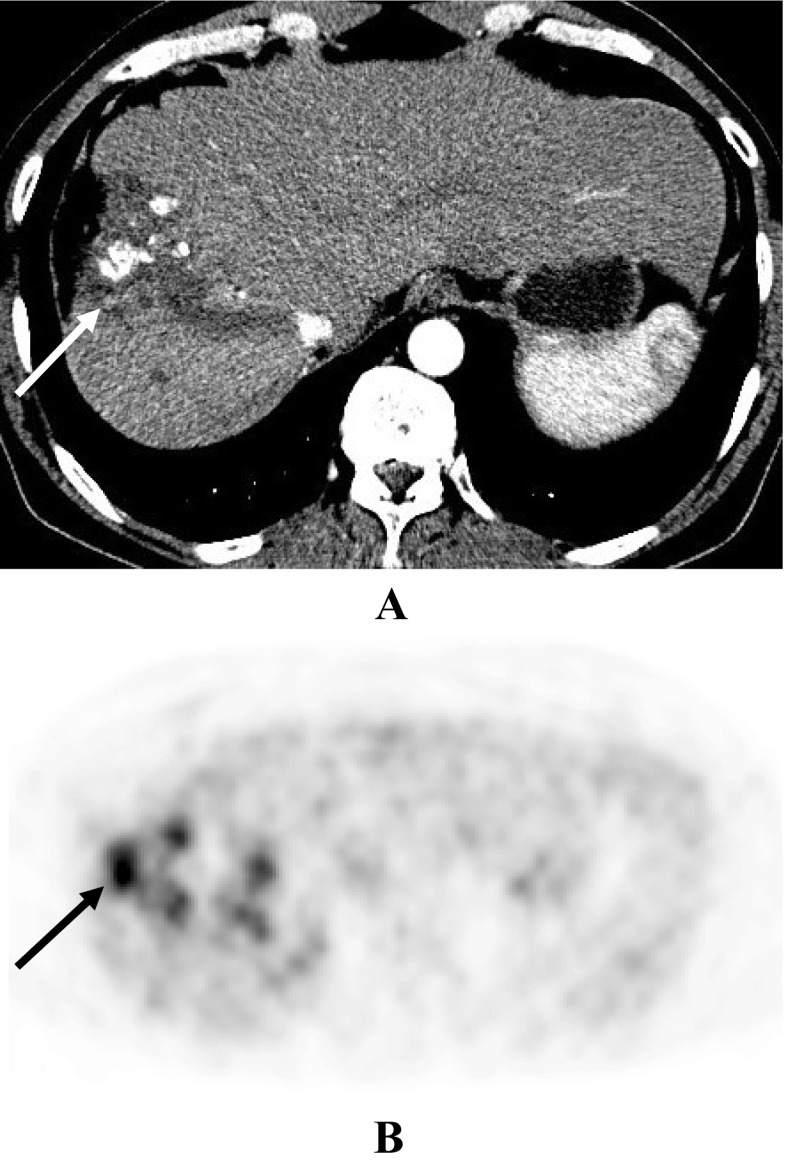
53-year-old man with partially necrotic HCC after TACE and placebo. (A), Transverse CT image (arterial phase) showing a subtotally necrotic tumor with low density masses and a focal hypervascularized tumor remnant (arrow) with patchy medium uptake; (B), Transverse [^18^F]-FDG PET showing high uptake of [^18^F]-FDG in the tumor remnant (arrow)
